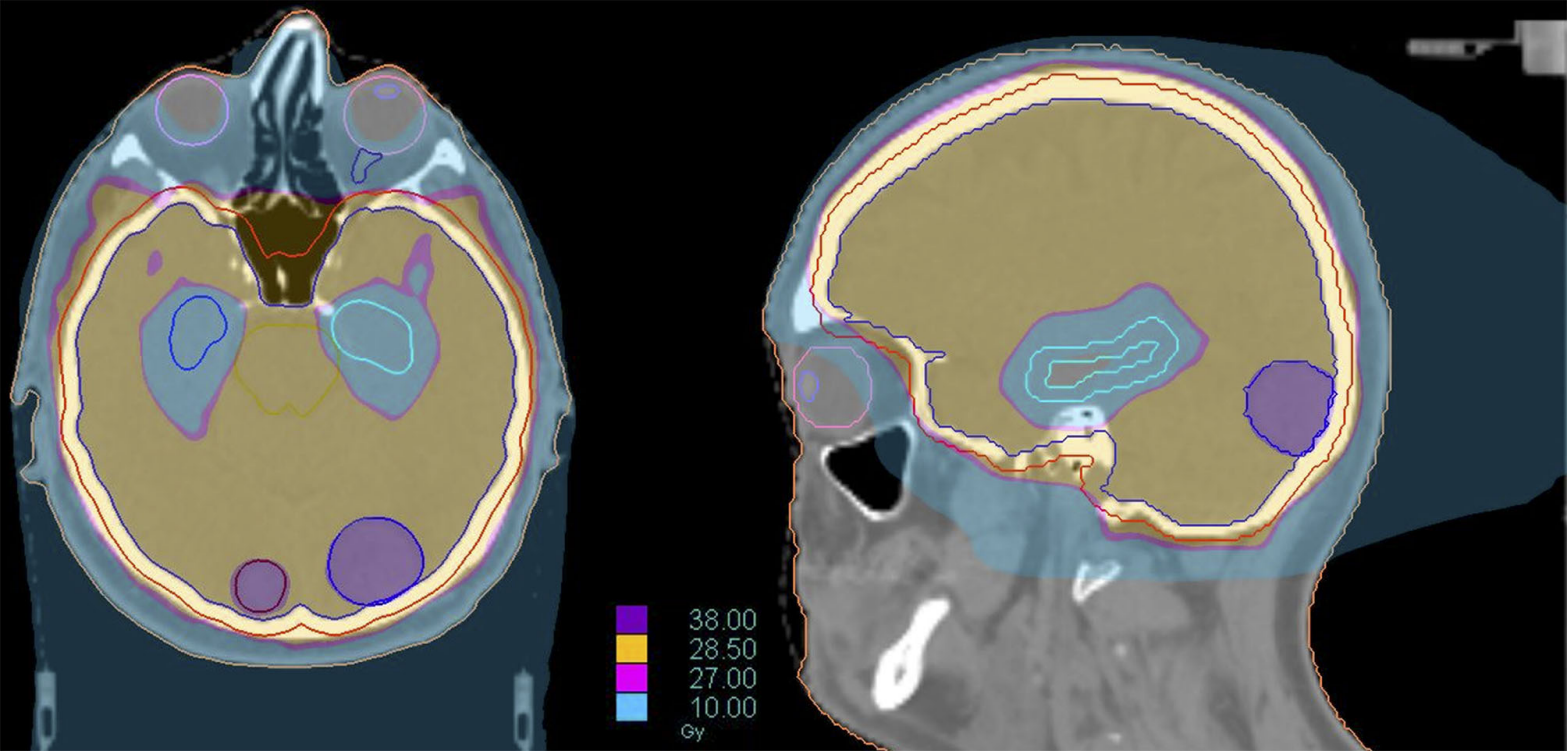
Dose distribution of a treatment of HA-WBRT plus SIB in patient with bilateral occipital BMs (left 2 cm; right 7 mm). The treatment was delivered with Helical tomotherapy and a daily control of the patient’s positioning with MV-CT. The dose delivered to the whole brain was 30 Gy in 10 fx with a concomitant boost of 40 Gy in 10 fx to the 2 lesions.
